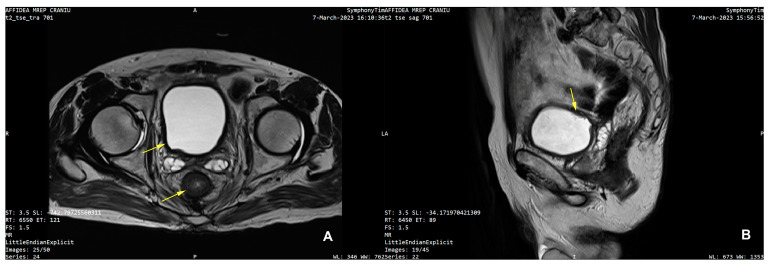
MRI re-evaluation post-chemotherapy (A) axial images:with arrows pointing regression of the bladder wall thickening and of the concentric rectal tumor; (B) sagittal view with arrow pointing downsized bladder tumor.
